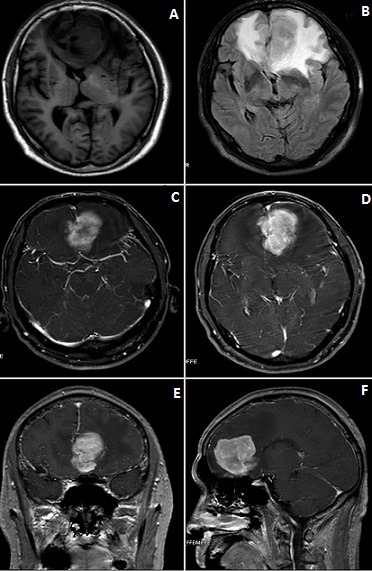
(A-F) are MRI showing the lesion: the lesion is located at bilateral frontal lobe and corpus callosum with massive vasogenic edema surrounding it
